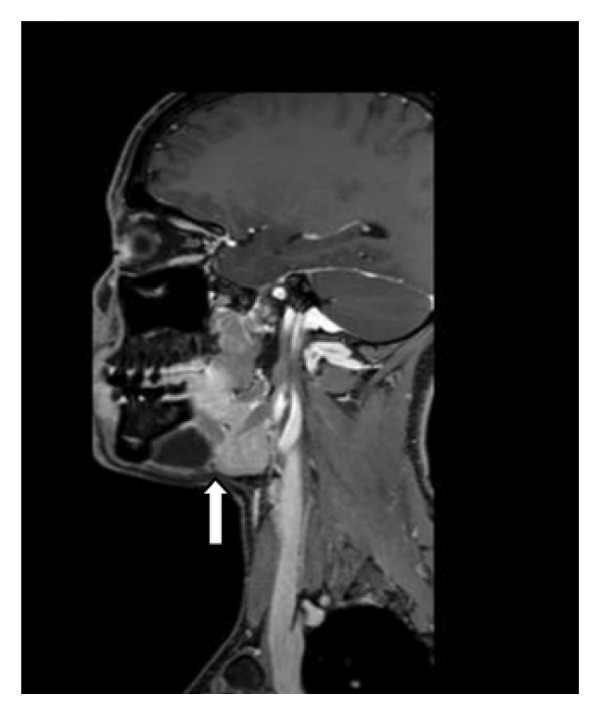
MRI sagittal images reveal bilateral cystic masses in the mandible, extending into the submandibular space. The masses exhibit well‐defined borders and show no enhancement.
